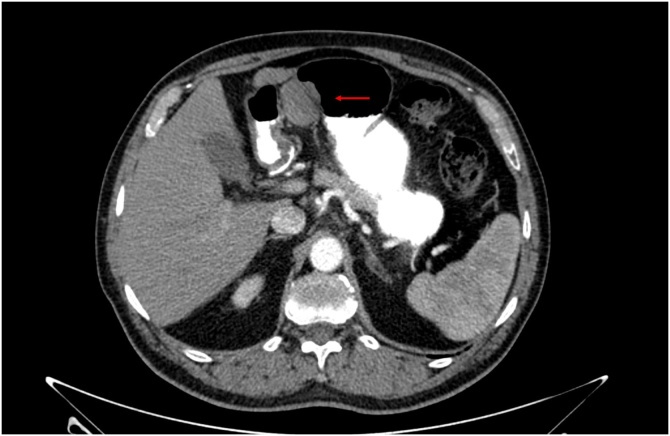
CT with oral and IV contrast. Image shows a mass arising from the lesser curvature of the stomach, which does not enhance with IV contrast.
